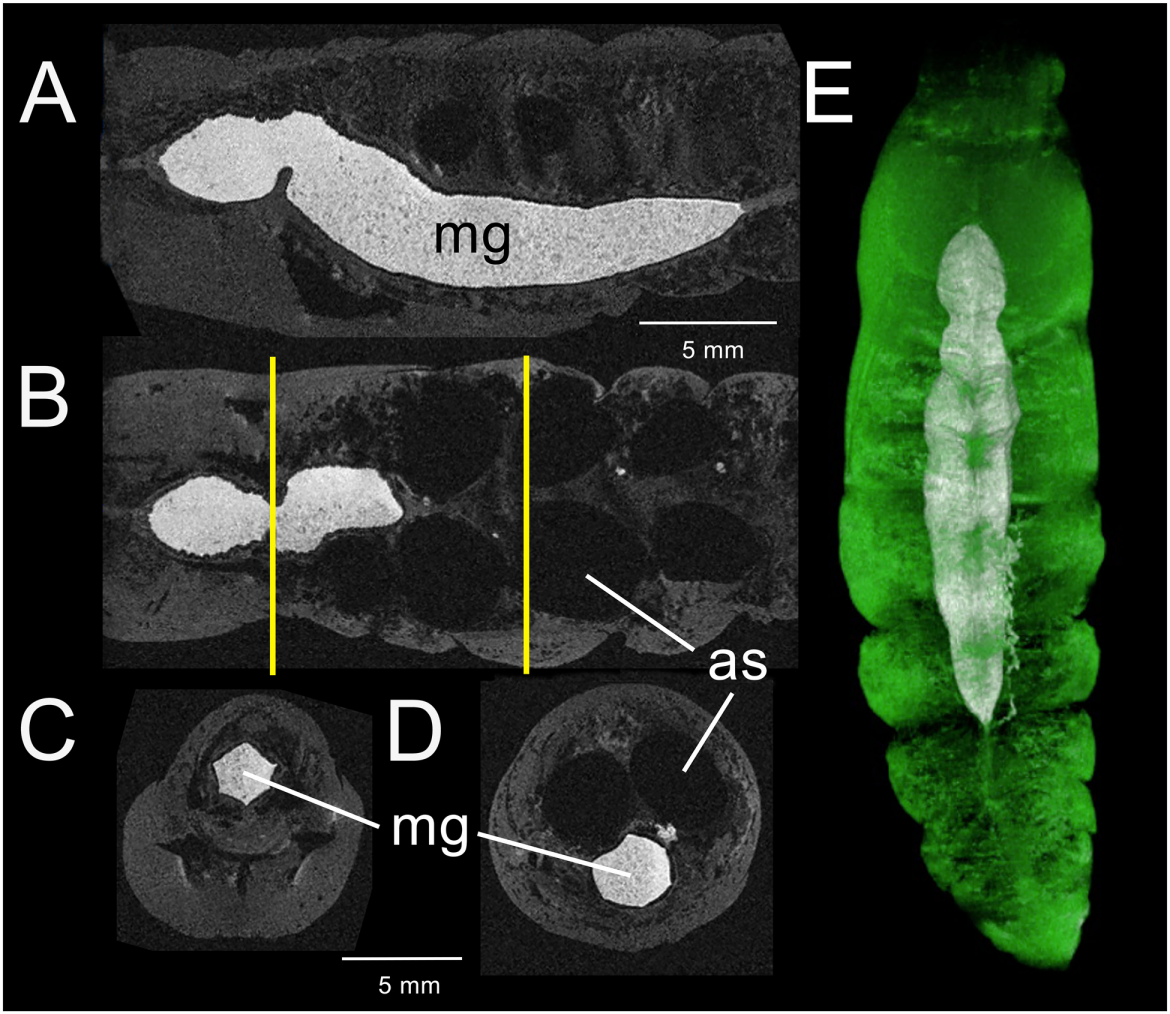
3D-GRE MR imaging data acquired from a *M*. *sexta* pupa 2–3 days after metamorphosis. (A) Sagittal view of thorax and abdomen displaying the enlarged midgut (mg) and newly formed air sacs (as). The contents of the midgut appear heterogeneous and lack gas bubbles. (B) Coronal view of the same segments, with air sacs prominent. (C) Transverse section through the thoracic-abdominal junction. Left line in (B) indicates level of section. (D) Transverse section through abdominal segment 5 displaying the enlarged air sacs that overlie the midgut. Right line in (B) indicates level of section. (E) Dorsal 3D surface-rendering showing the CA-enhanced midgut together with remnants of the right silk gland. Green regions overlying the midgut represent heart chambers.
